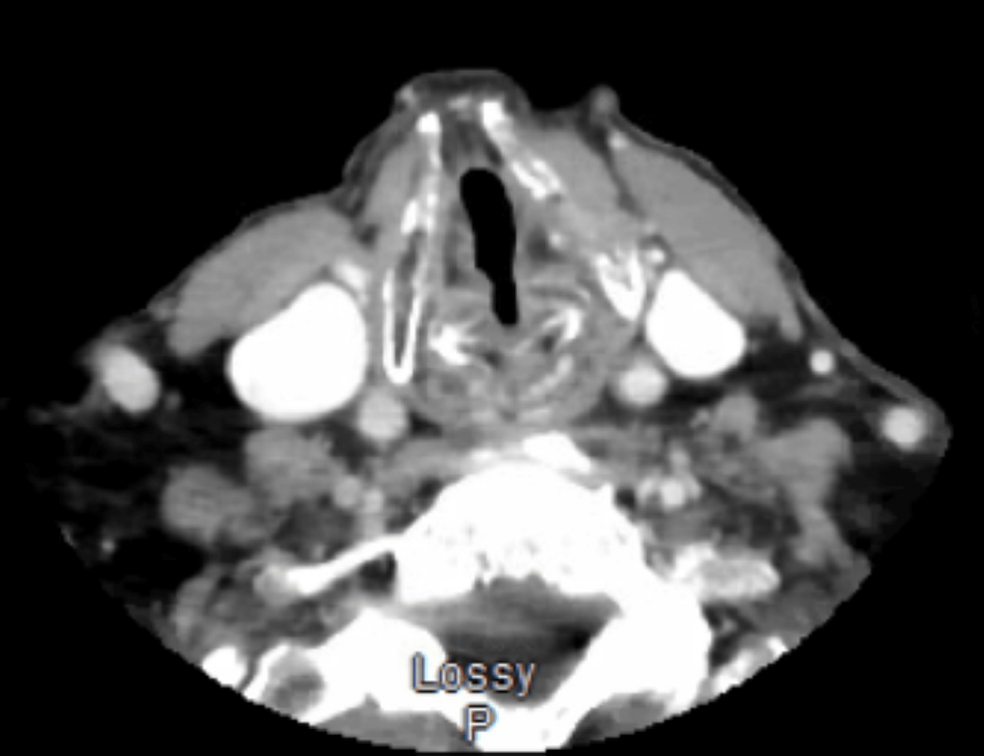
Repeat CT scan, which was interpreted by a radiologist as no evidence of vocal fold mass. CT, computed tomography.
